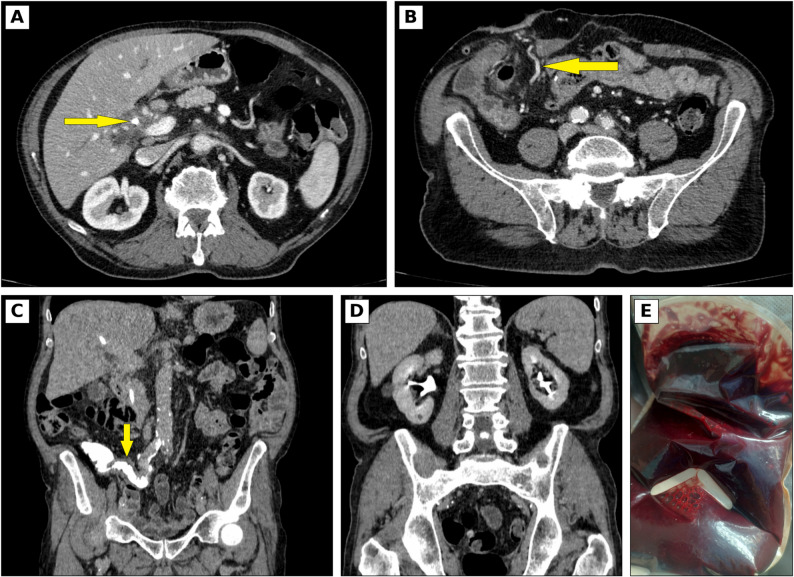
Imaging and clinical appearance of conduit hemorrhage. A Axial portal venous-phase computed tomography showing the biliary stent and periportal or pancreatic tumor-related changes. B Axial portal venous-phase computed tomography showing venous collaterals adjacent to the Bricker conduit. C Coronal urographic-phase reconstruction showing circumferential thickening of the conduit wall. D Coronal urographic-phase reconstruction showing nondilated upper urinary tracts without radiologic evidence of recurrent urothelial carcinoma. E Urostomy bag filled with gross blood and clot during the index bleeding episode
